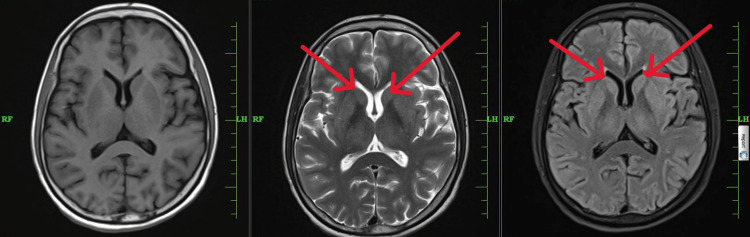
T1- and T2-weighted MRI of the brain shows subtle hyperintensities in the bilateral caudate lobe (red arrows) MRI: Magnetic resonance imagining; A: T1-weighted MRI of the brain; B: T2-weighted MRI of the brain; C: T2 FLAIR MRI of the brain
